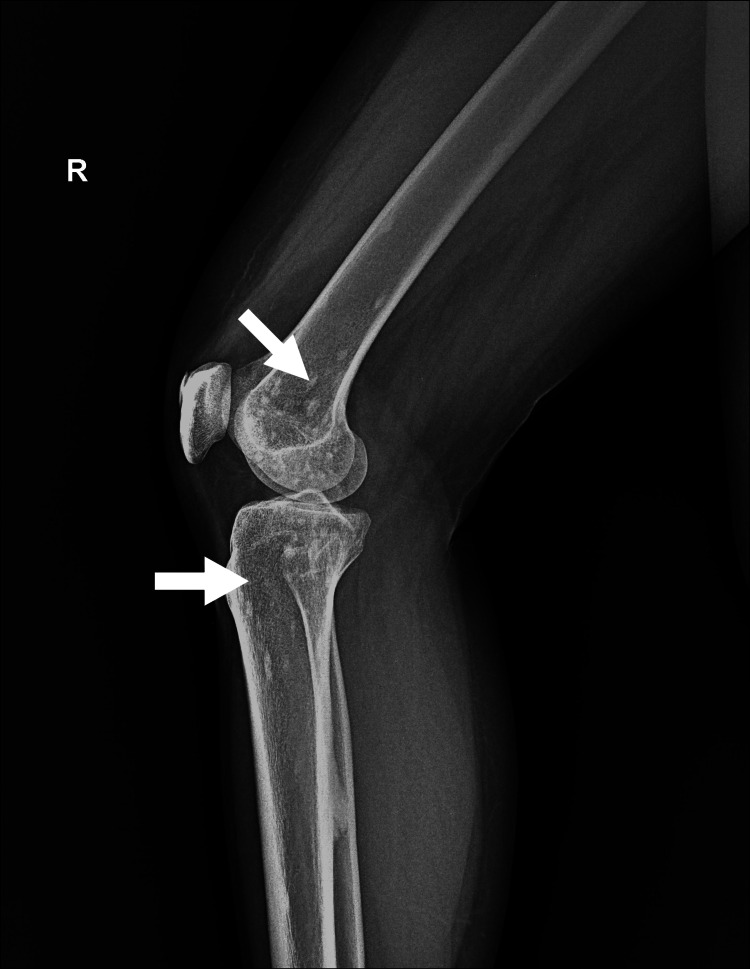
X-ray lateral view of the right knee White arrows represent the multiple bony lesions in both the femur and tibia. R: right lower limb
